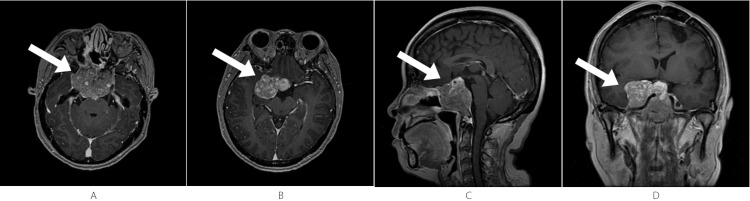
Pre-IMPT - October 2023 The lesion involves the clivus and bilateral cavernous sinuses, encasing both internal carotid arteries. The lesion compresses the brainstem, and the optic chiasm is compressed and displaced superiorly. Both optic nerves are encased by the lesion. (A, B) Axial T1-weighted post-contrast images show a sellar mass with heterogeneous enhancement (arrow). (C): Sagittal T1-weighted post-contrast image shows the lesion with heterogeneous mild-to-moderate enhancement (arrow). (D) Coronal T1-weighted post-contrast image shows the lesion with heterogeneous mild-to-moderate enhancement (arrow). IMPT, intensity-modulated proton therapy
